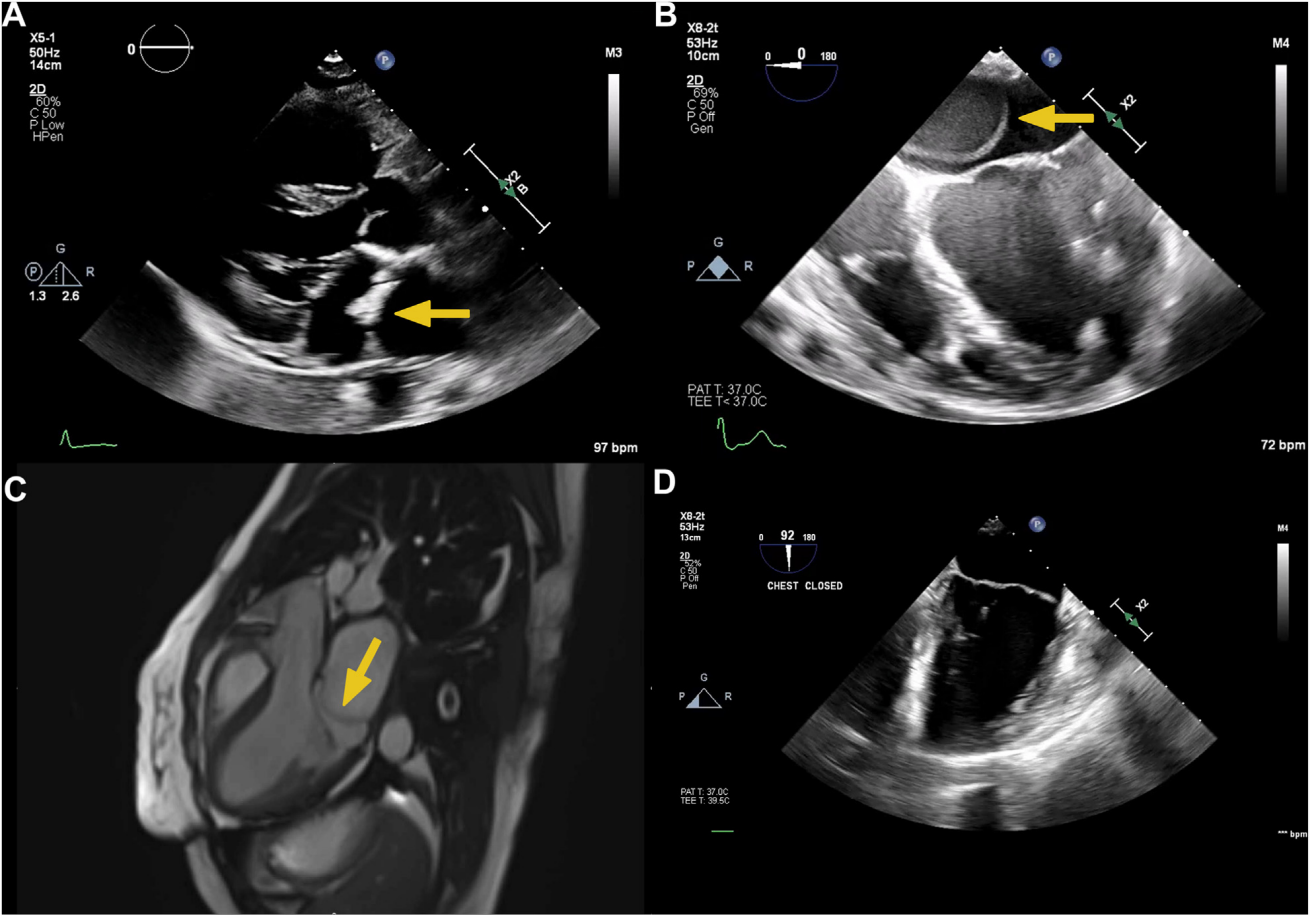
Multimodality comparison images. (A) Two-dimensional transthoracic echocardiography, parasternal long-axis systolic view, (B) two-dimensional TEE, midesophageal four-chamber (0°) low-quality (overgained), systolic view, (C) CMR, balanced steady-state free precession sequence, three-chamber diastolic display, and (D) postoperative two-dimensional TEE, midesophageal two-chamber (92°) systolic view, demonstrate the fibromuscular division of the left atrium proximal to the MV plane *(arrows)*, consistent with the CTS membrane and the normal left atrium and MV after resection.
